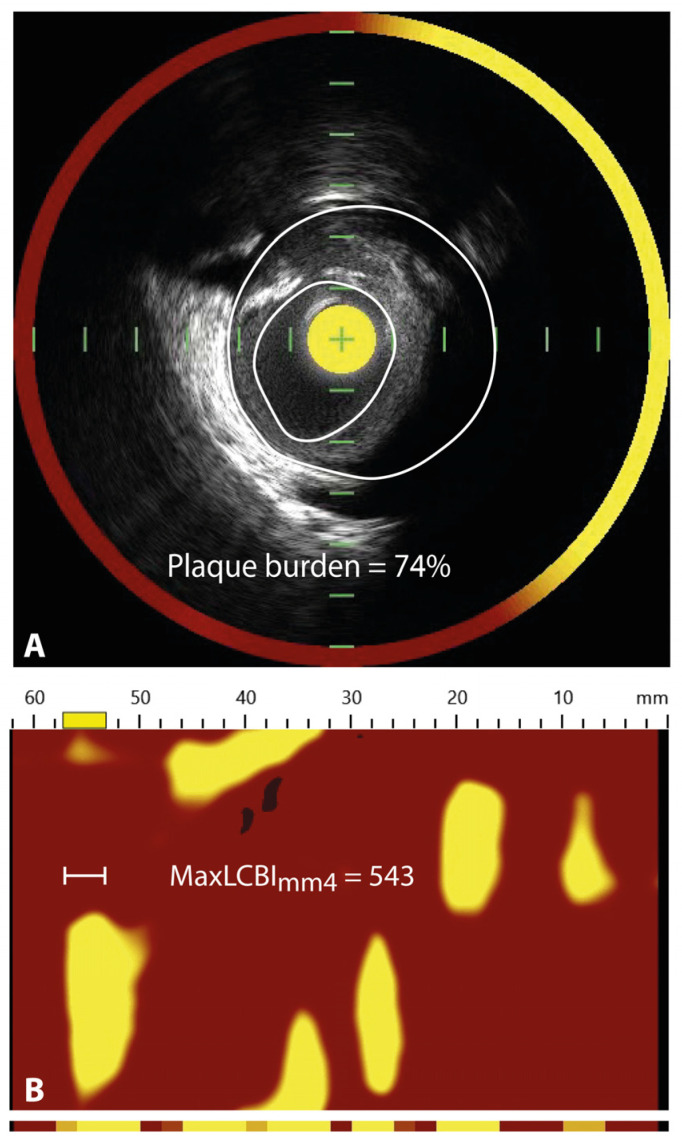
Vulnerable plaque on NIRS-IVUS. (A) displays an intravascular ultrasound (IVUS) image, which demonstrates an echolucent plaque with deep echo attenuation and a large plaque burden of 74%. The red-to-yellow colored ring corresponds with near-infrared spectroscopy (NIRS) data. The ring colors yellow at the site of the soft plaque, indicating that the plaque corresponds with a high probability for lipid core. In (B), the corresponding NIRS chemogram is displayed, where a maximum lipid-core burden index in a segment of 4 mm (maxLCBImm4) is detected of 543 at around 55 mm of the pullback, corresponding with the definition of a lipid-rich plaque (maxLCBImm4 > 400), based on the LRP study [79]. Image adapted from Van Veelen et al. Reviews in Cardiovascular Medicine 2022. CC-BY [4.0] [9].
